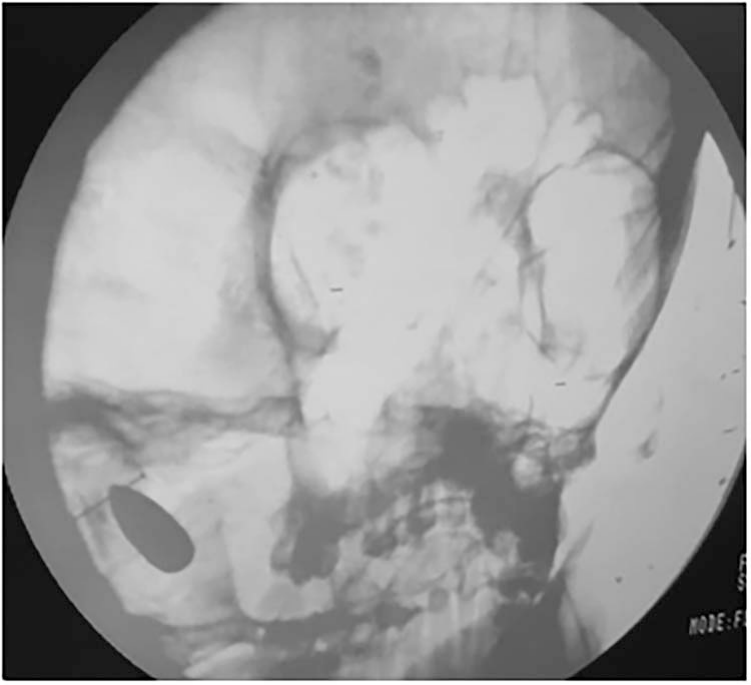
Intra-operation skull X-ray revealed that bullet had inversed and migrated upwards inside the left cerebellar hemisphere.
